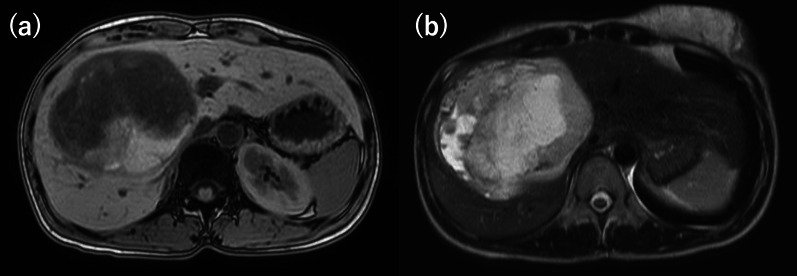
a Magnetic resonance imaging reveals a hypointense multicystic tumor on T1-weighted and b hyperintense on T2-weighted images
